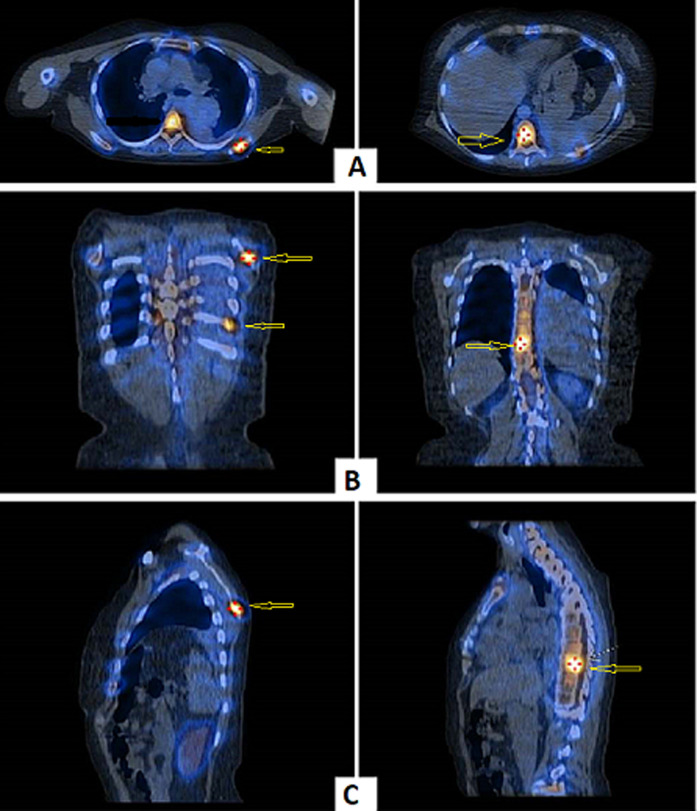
functional single-photon emission computed tomography images in axial view (A), coronal view (B), and sagittal view (C) showing the increased area of activity in the inferior angle of the scapula, 10^th^ left rib posteriorly, and the vertebral discs suggestive of metastasis (arrows)
